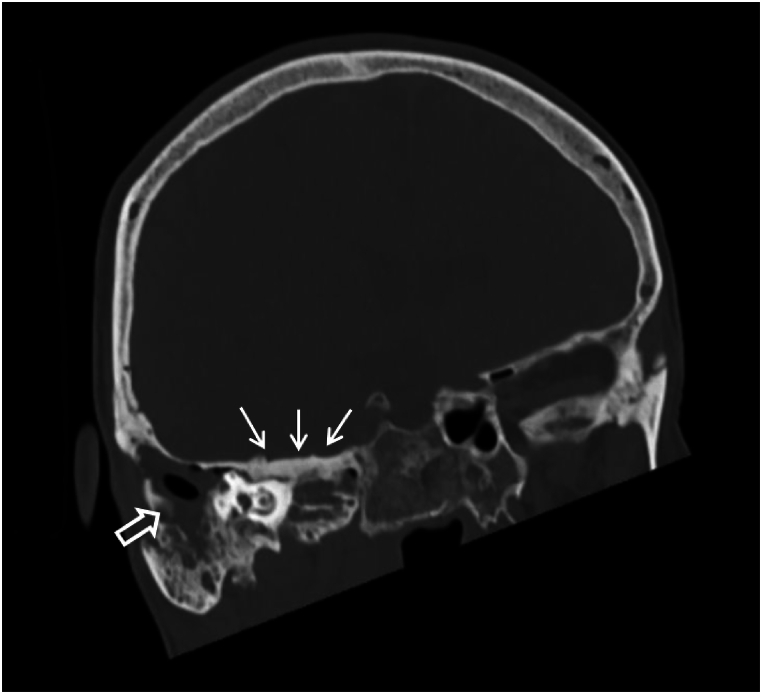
Coronal oblique reconstructed CT slide showing hyperostosis of the right temporal bone (arrows) as part of the intra-osseous meningeoma. There is a postoperative state with an opacified mastoid cavity (open arrow) and intact tegmen tympani.
